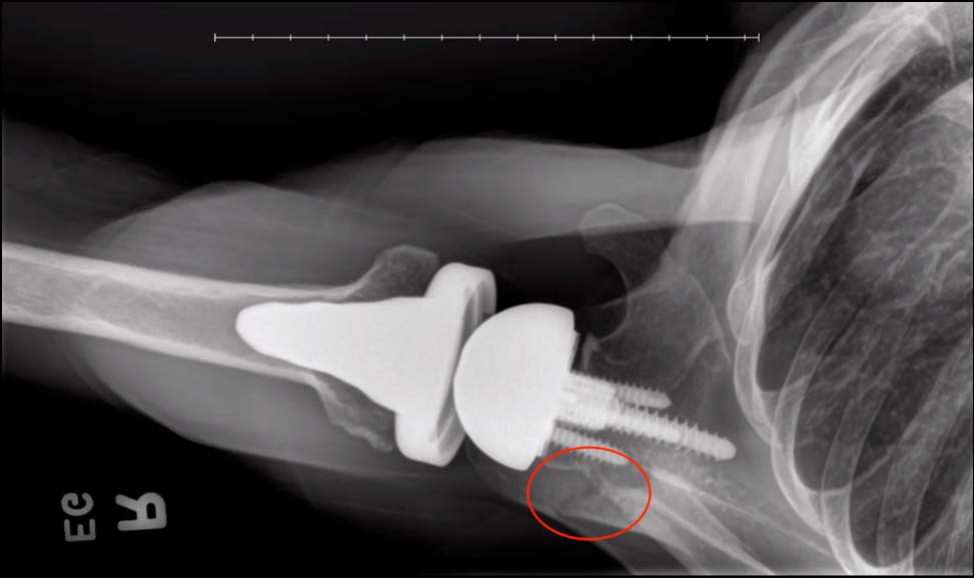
Radiograph of patient 1 at presentation of an acromial fracture, Levy type II.
